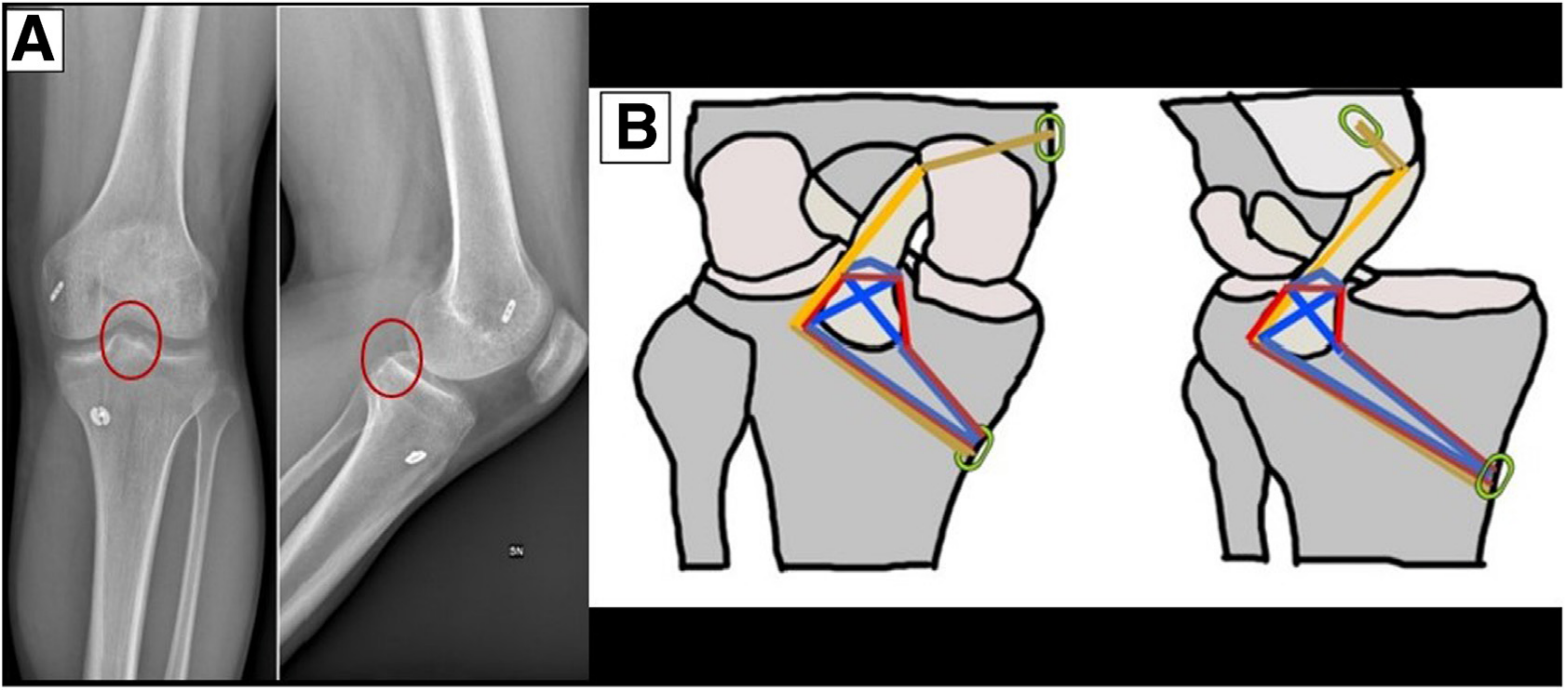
Left knee. Postoperative radiograph and summary of suture fixation technique. (A) The postoperative radiograph shows successful reduction and fixation of the avulsion fracture of the posterior cruciate ligament (PCL) using our procedure. The RT button (Arthrex) can be seen fixed to the medial femoral cortex, whereas the ABS button sits on the medial tibial cortex. Both the anteroposterior and lateral projections show excellent fracture reduction (ovals). (B) Summary of arthroscopic all-inside PCL avulsion fracture suture fixation with double-tunnel pullout and InternalBrace augmentation. The red suture represents the 1.5-mm high-strength ribbon suture (LabralTape), configuring the trans-cruciate suture; the blue suture represents the high-strength, nonresorbable suture tape (FiberTape) that embraces the PCL remnant from anterior to posterior and is configured in a crosstie fashion. The gold suture represents the InternalBrace ligament augmentation (IBLA) system. The IBLA is secured with a loop-adjustable cortical fixation device (TightRope RT) on the femoral side (green ovals) and is retrieved through the lateral tibial tunnel with the PCL sutures in the posterolateral (PL) portal. The tips of the sutures and the IBLA are secured onto an ABS button on the tibial side (green ovals).
